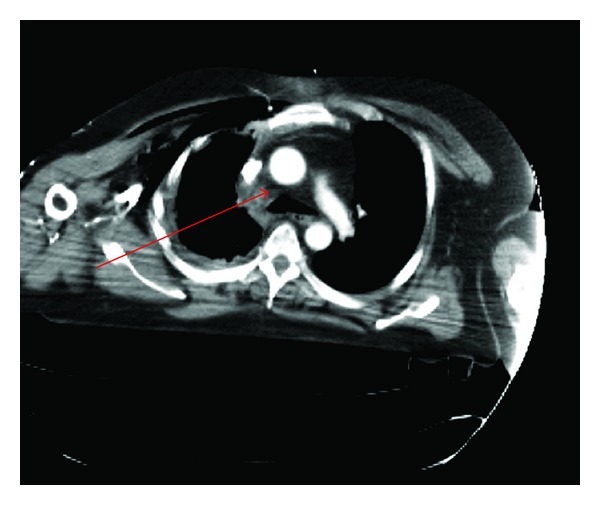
CT Thorax with a right-sided paratracheal haematoma at the level of the azygous vein (see arrow).
